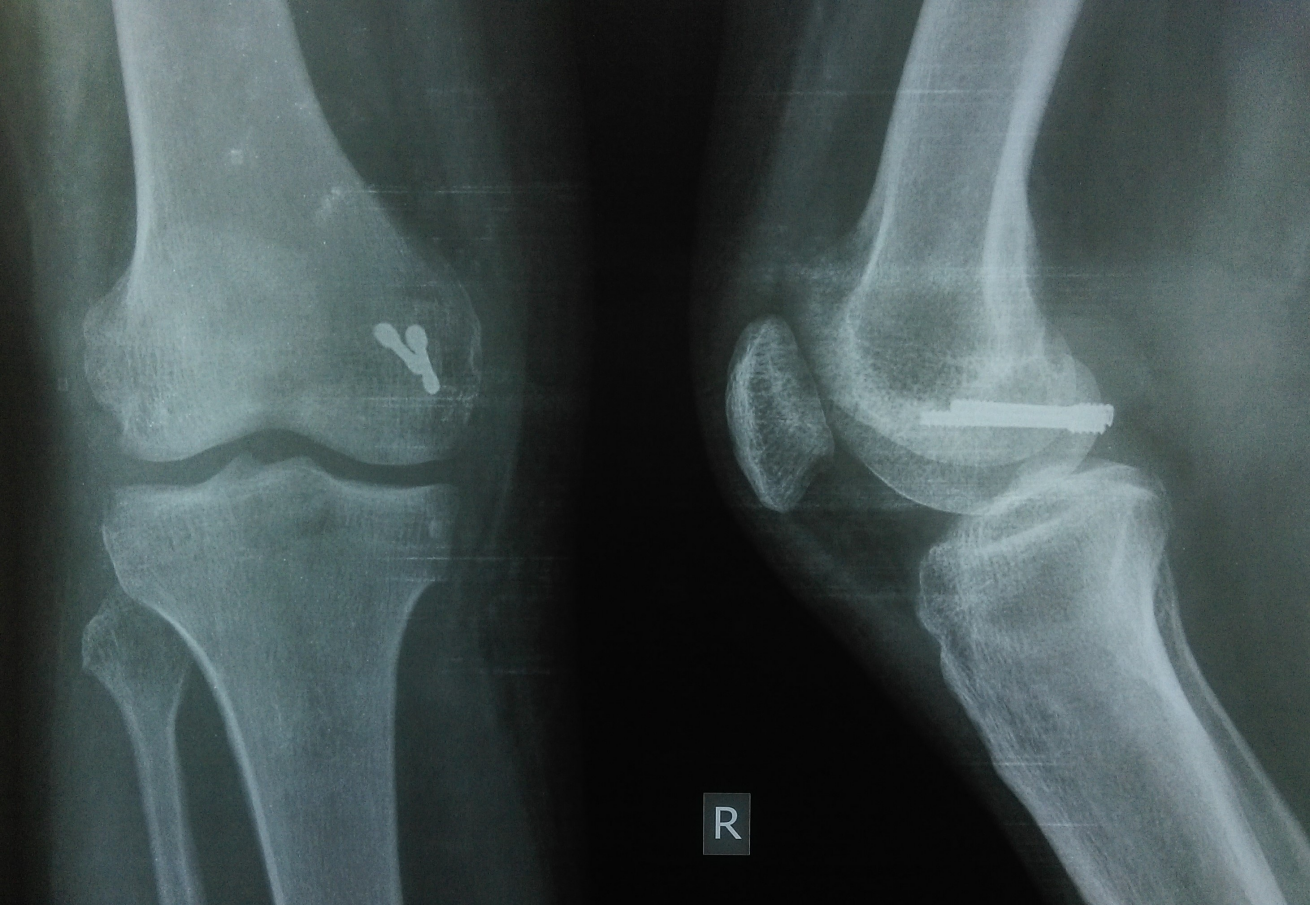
A 10-week follow-up Xray image of the anteroposterior and lateral views showing the union of the fracture with in-situ Herbert screws
